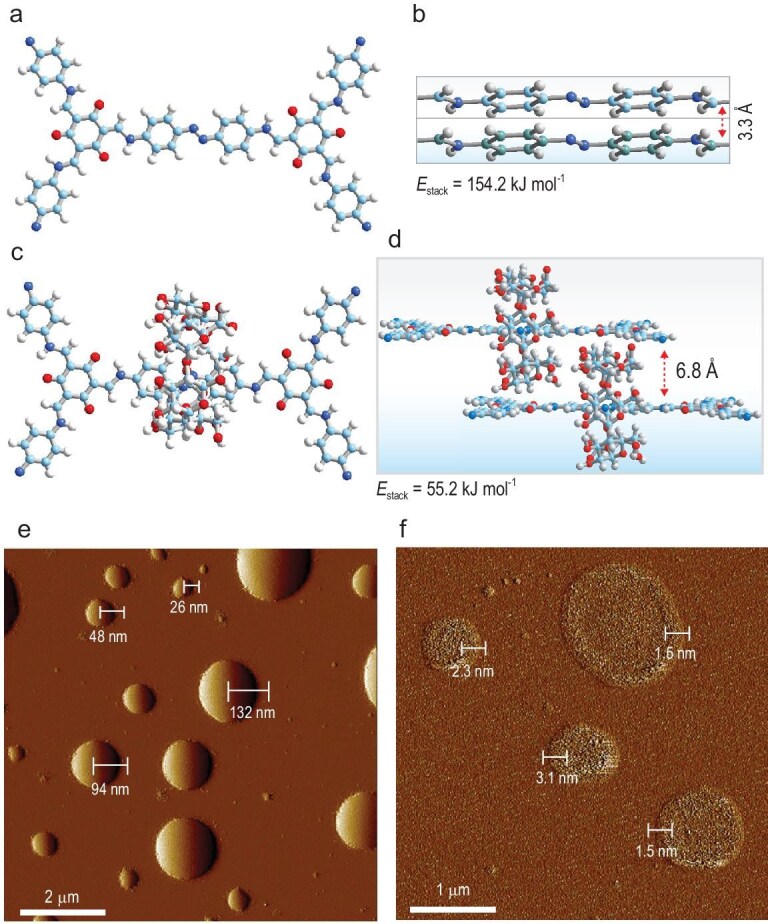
Stacked 2D layers of TpAzo ((a) top view and (b) side view) and CD-TpAzo ((c) top view and (d) side view), and the AFM thickness images of (e) TpAzo@H_3_PO_4_-13 and (f) CD-TpAzo@H_3_PO_4_-18 after ultrasound in water.
